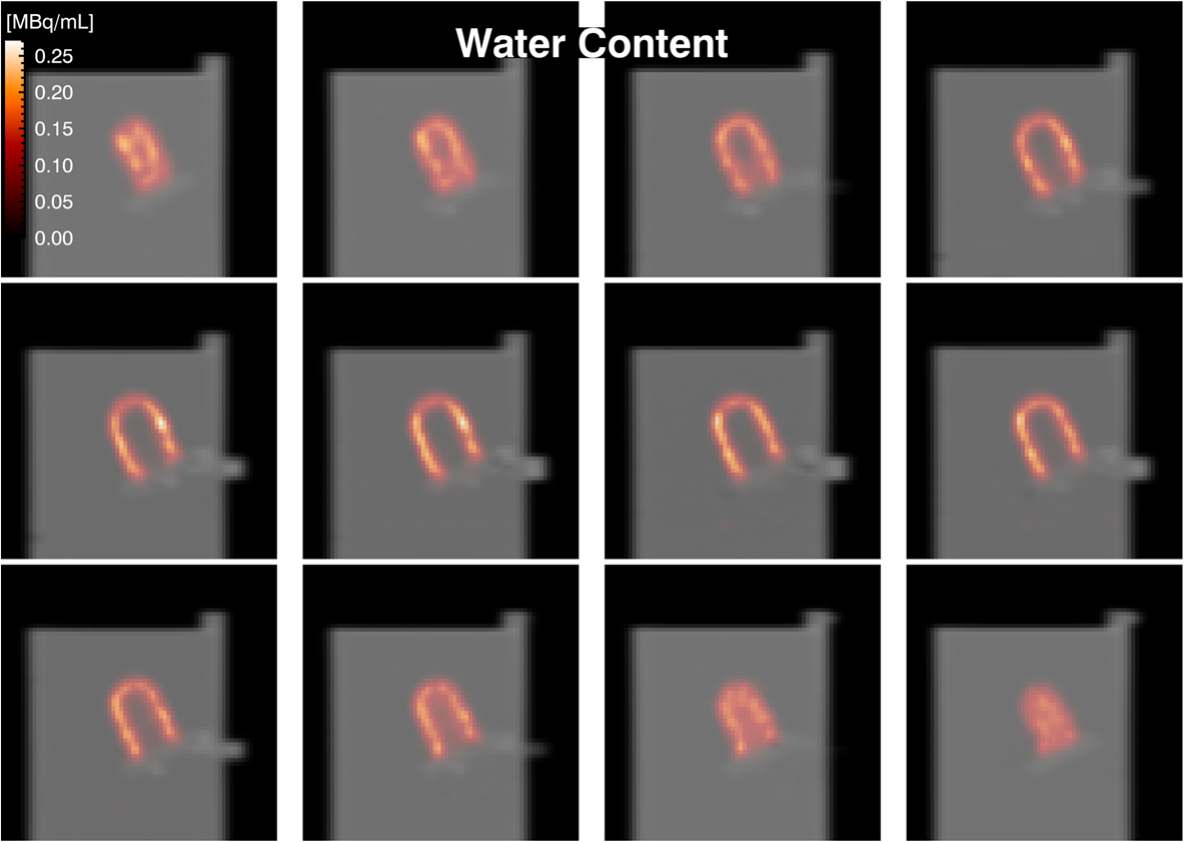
Twelve consecutive SPECT slices (50 iterations) overlaid on the corresponding CT slices for a cardiac insert in a water-filled torso. The ventricle cavity was filled with water. The MCR and CT results are well registered with good contrast between the myocardium and ventricle. Despite the attenuation in the cavity water, the homogeneity of the activity in the myocardium is well recovered, albeit somewhat nosier than in Fig. 7, due to the modelling of attenuation in the forward-projector
